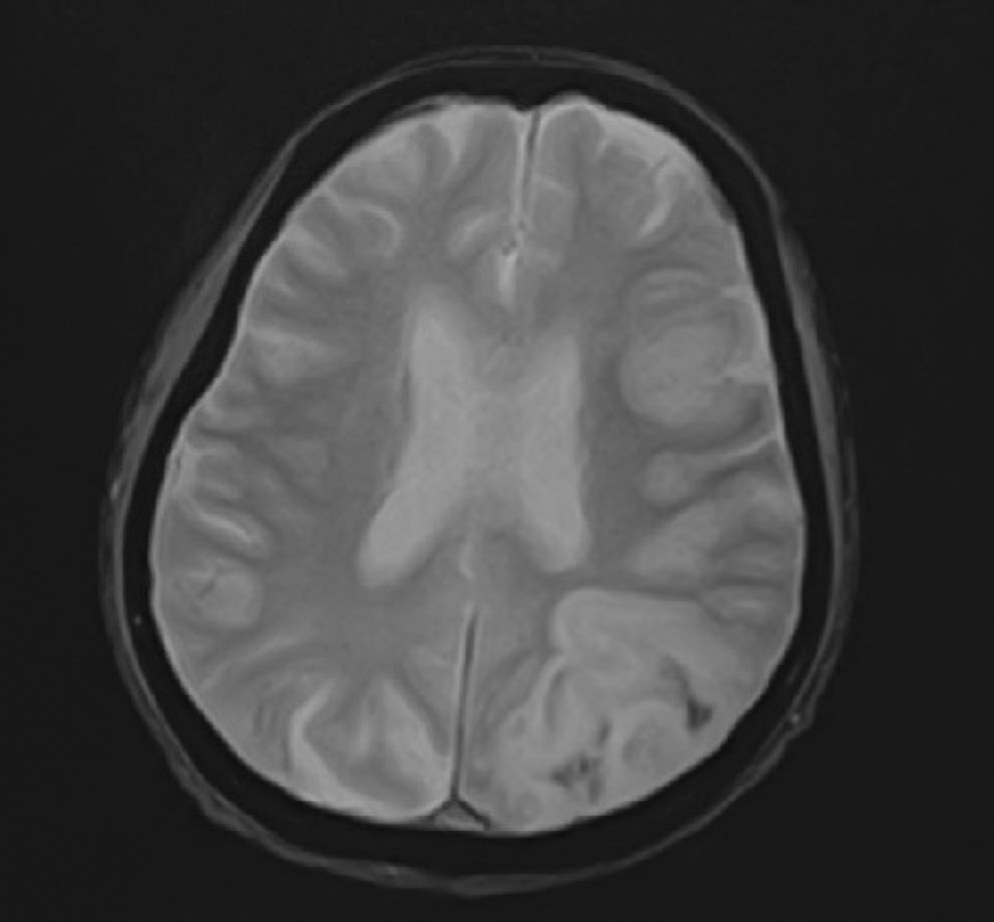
FIGURE 3 MRI axial SWI image
